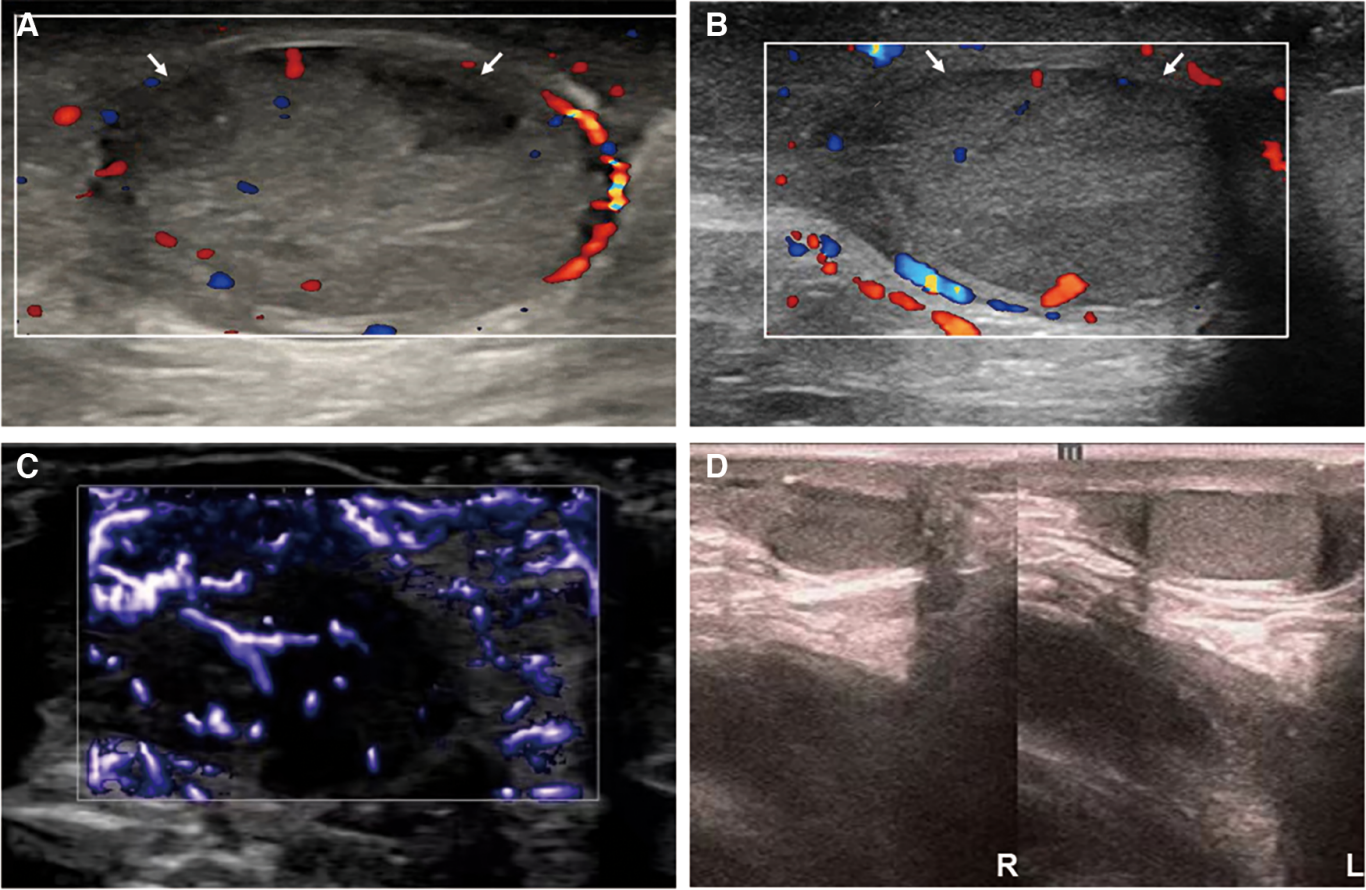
Ultrasound images of the right testicle during treatment and follow-up. From postoperative day 3 (A) to postoperative week 2 (B), the ultrasound showed two patchy hypoechoic areas (arrow) in the right testicle, which gradually narrowed and almost returned to normalcy. Two weeks after surgery, testicular ultrasound microvascular imaging (C) showed a short lines-like blood flow signal in the right testicular tissue. The ultrasound follow-up conducted 3 months after surgery showed that the right testicle was slightly smaller than the left testicle (D).
